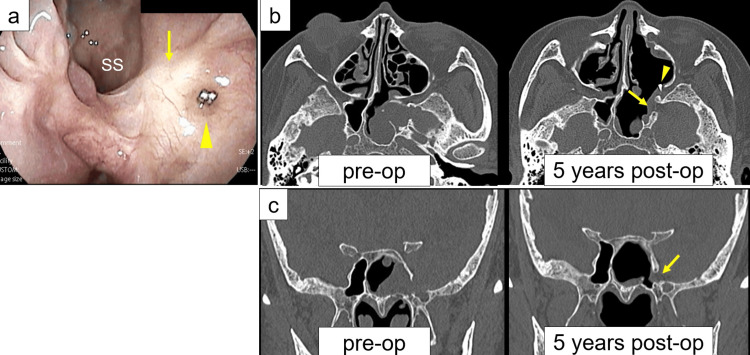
Findings at five-year follow-up after surgery. (a) Nasal endoscopy five years after surgery showing epithelialization of the cranial base reconstruction site, with vascular clips visible on the mucosa. (b) Axial HRCT views before (left; also shown in Figure 1b) and after (right) surgery, demonstrating reconstruction of the skull base defect with no recurrence. (c) Coronal HRCT views before (left; also shown in Figure 1c) and after (right) surgery, confirming stable reconstruction with no recurrence of the meningocele. Preoperative images are presented alongside postoperative images for direct comparison. The arrows on panels (a-c) indicate the reconstruction site. The arrowheads on panels (a) and (b) indicate vascular clips. SS, sphenoid sinus
